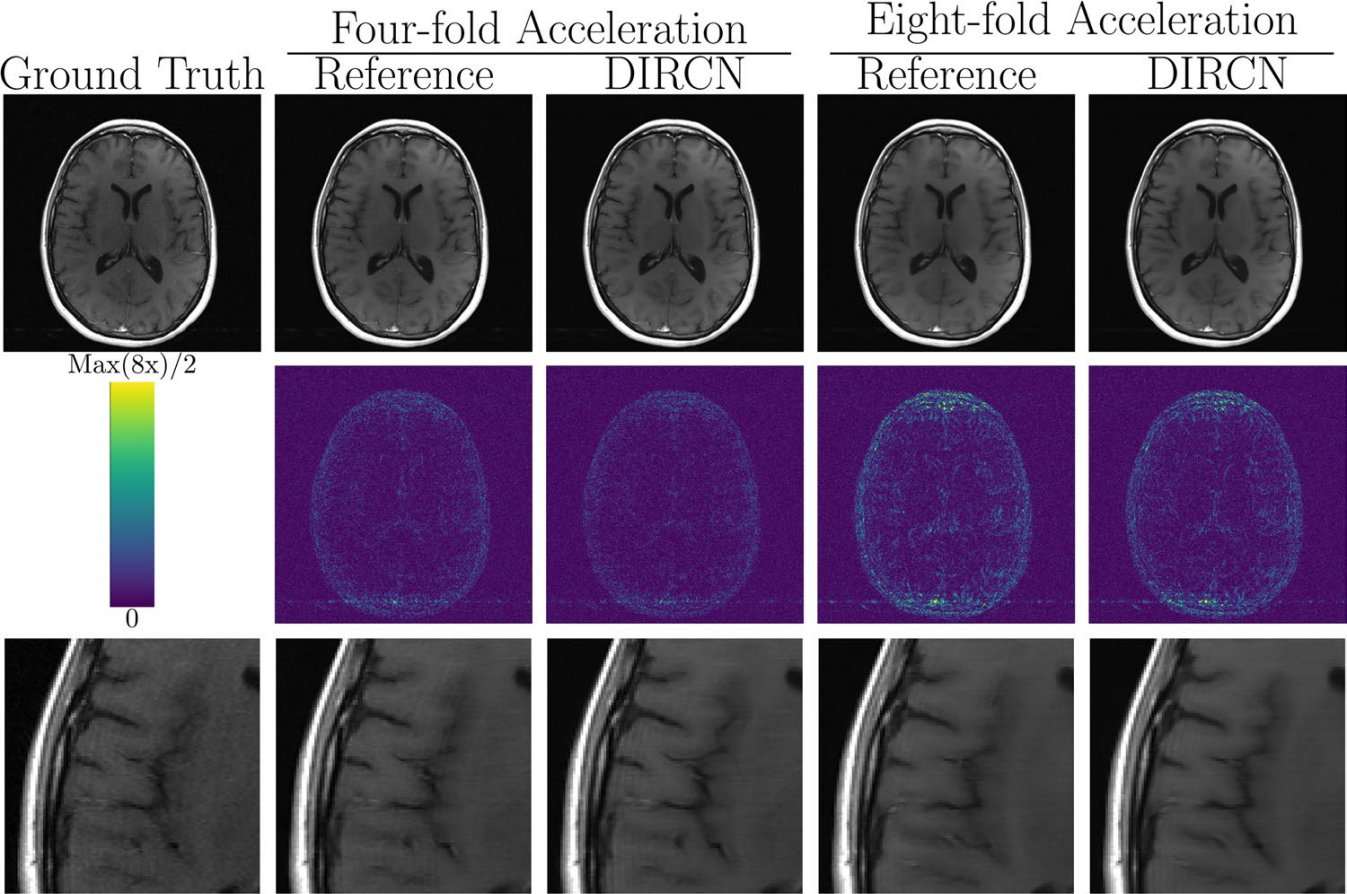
A representative example of a T1-weighted reconstruction with the reference model and DIRCN for four- and eightfold acceleration. This includes their respective reconstructions and the corresponding error map (absolute difference) between the fully sampled image and the reconstruction. The colormap goes between 0 and half the maximum error for eightfold acceleration to emphasize visual difference. The bottom images are a region of interest where slight improvement between the reference and DIRCN can be seen at close inspection
